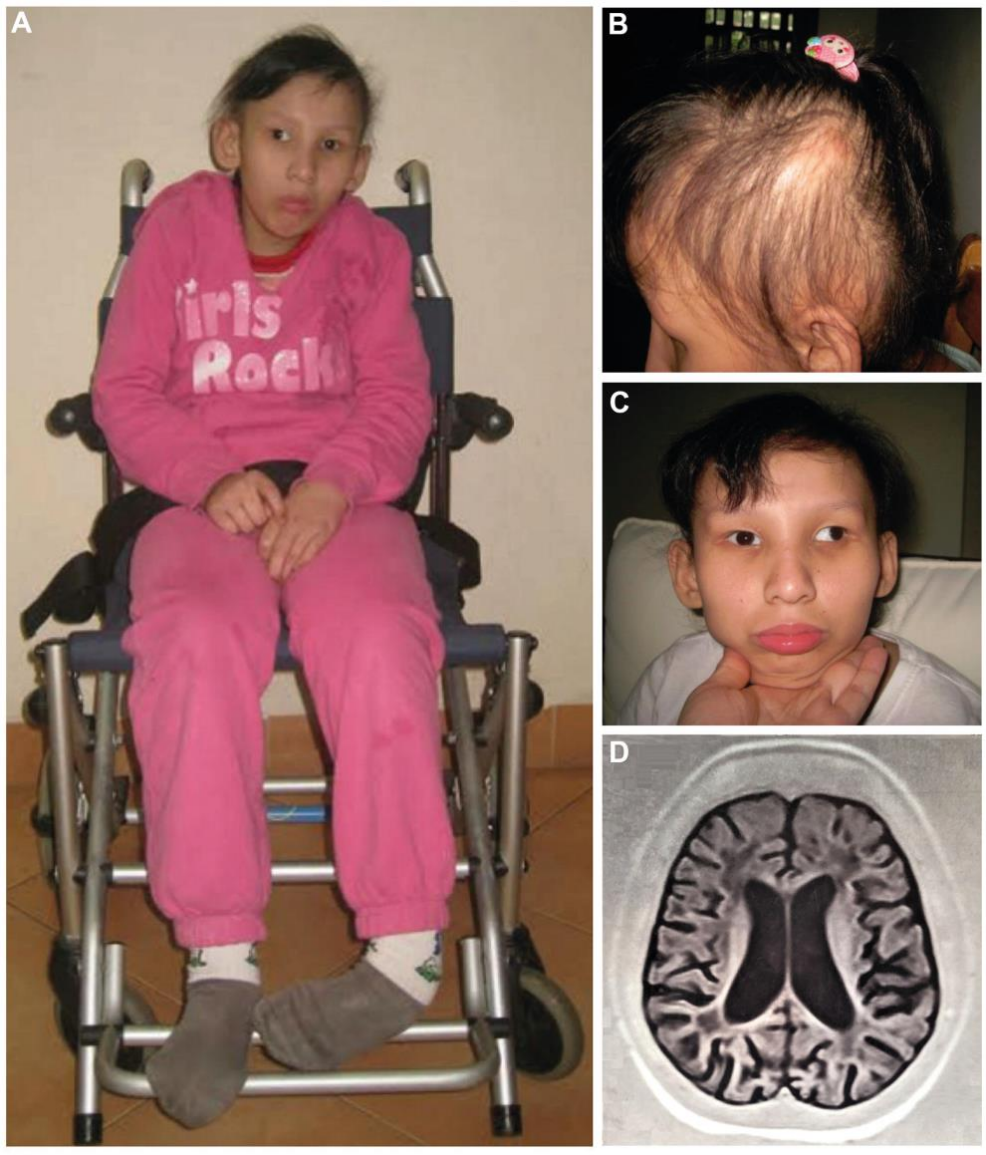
Proband at the age of 16 (A–C): (A) Full length figure of the proband, thinning hair (B) and strabismus at front vision (C) were observed. Brain T1-weighted MRI image at the age of 14 years (D).
